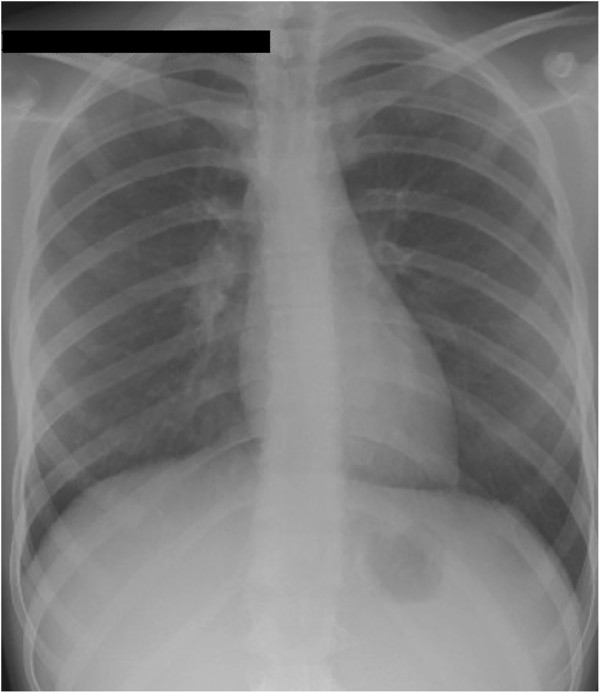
A postero-anterior chest radiograph was normal.
